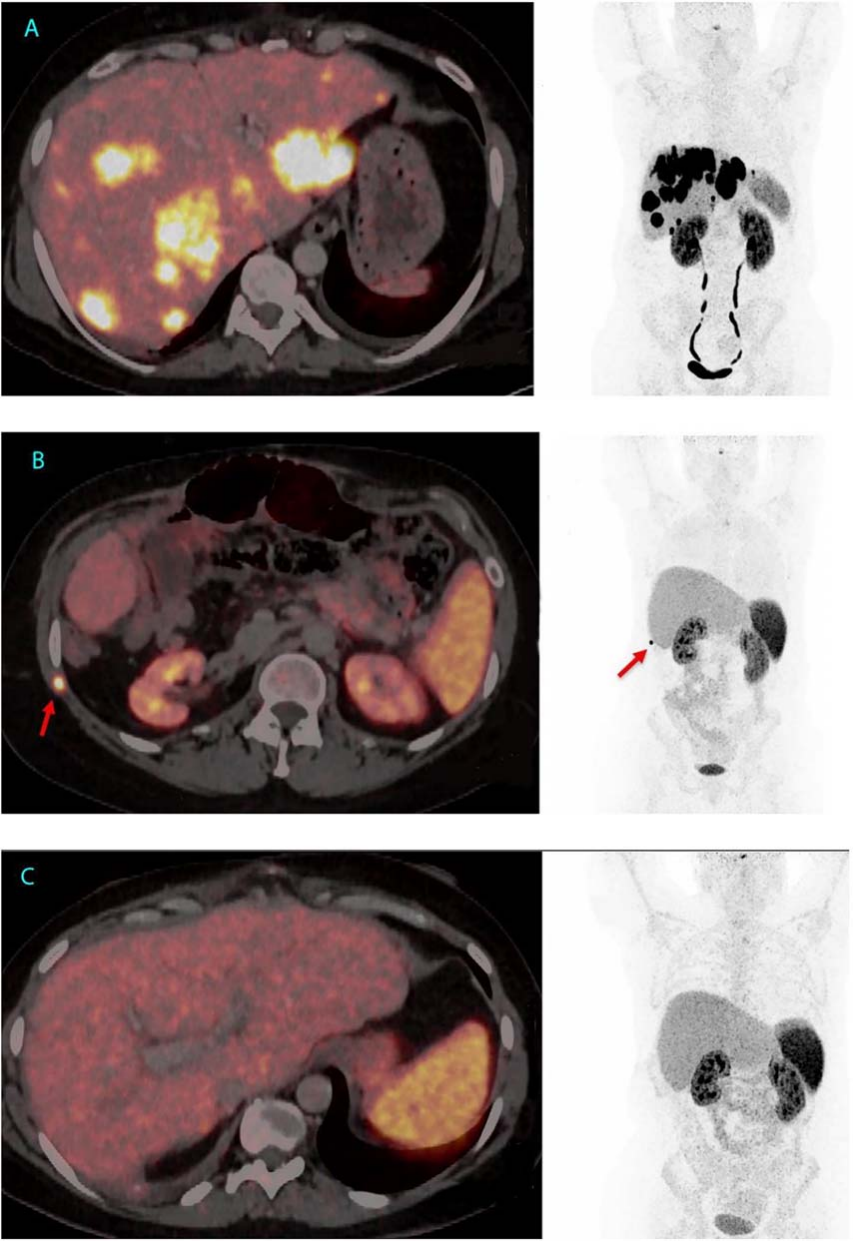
^64^Cu-DOTATATE PET/CT from 2 June 2022, 7 weeks before LT with extensive hepatic involvement but no extrahepatic metastases (A). ^64^Cu-DOTATATE PET/CT 15 months after LT revealed an implantation metastasis in the thoracic wall (B). Latest ^64^Cu-DOTATATE PET/CT performed 18 months after LT and 3 months after resection of the thoracic metastasis showed no signs of recurrence (C).
